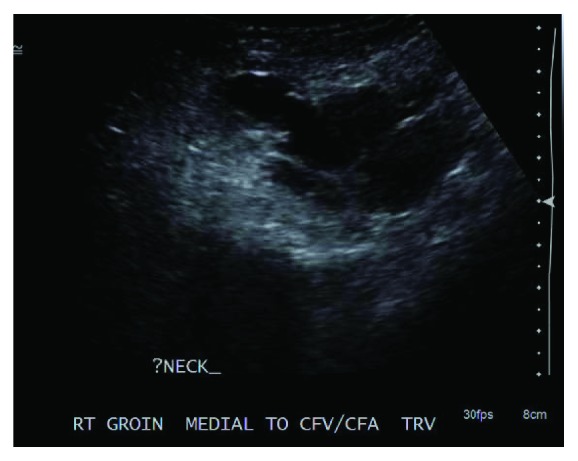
Duplex Ultrasound of the right common femoral artery pseudoaneurysm after the first thrombin injection.
